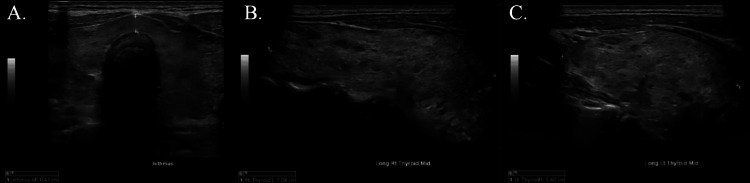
Thyroid ultrasound (A) Transverse image of the thyroid gland. (B) Longitudinal image of the left thyroid measuring 5.60 cm in length, showing enlargement with diffuse heterogeneous echotexture. (C) Longitudinal image of the right thyroid measuring 7.08 cm in length, showing enlargement with diffuse heterogeneous echotexture.
